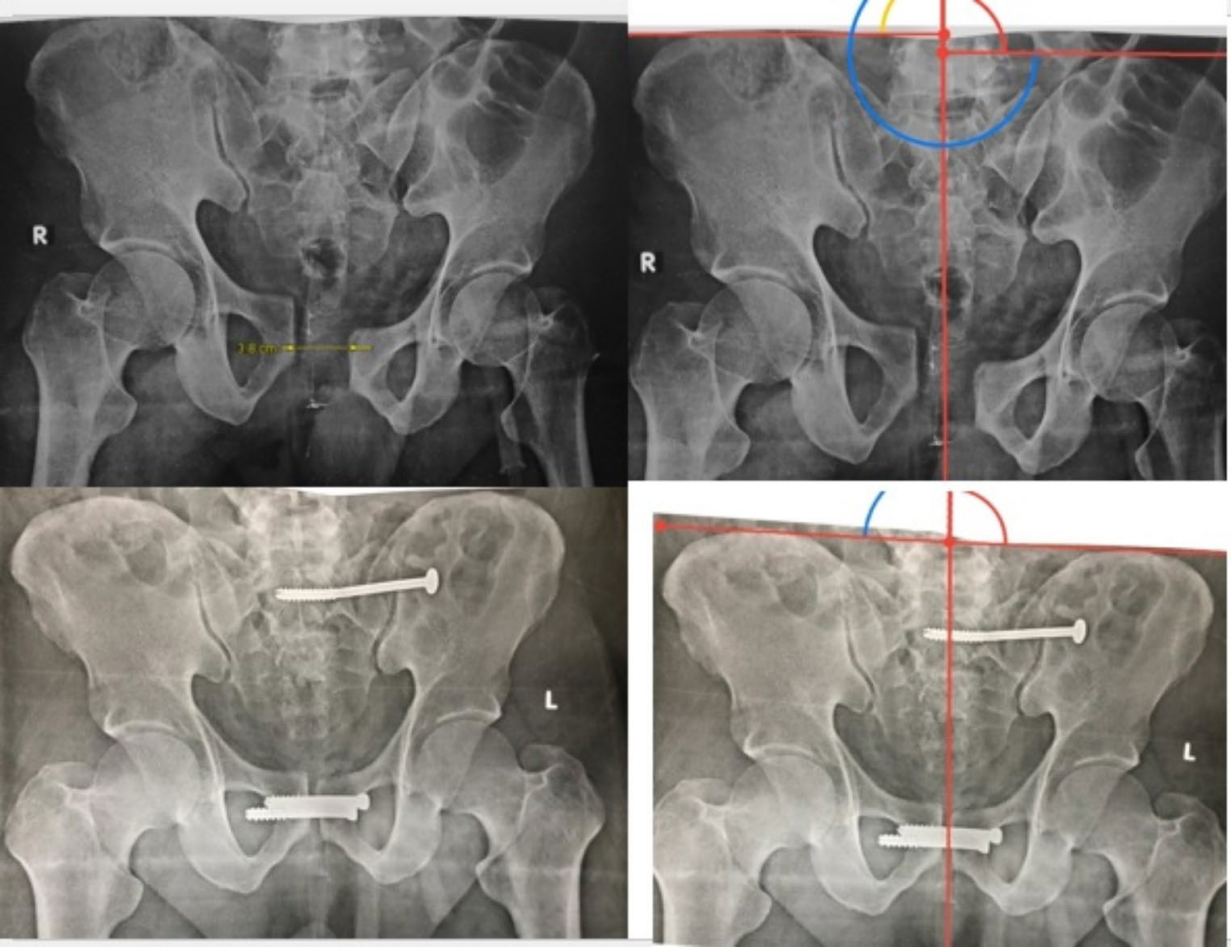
Preoperative and postoperative plain radiographs showing symphyseal screw fixation with measurement of vertical pelvic displacement using Henderson horizontal reference lines
